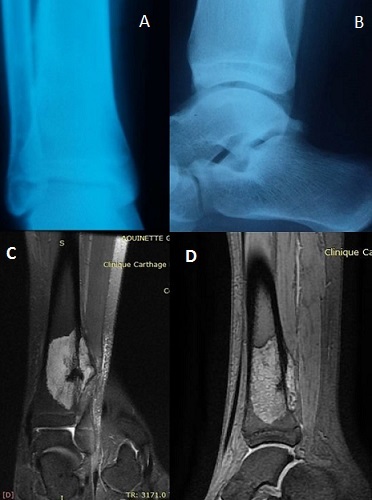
(A,B) plain radiographs showing a metaphyseal osteolytic lesion of the distal left tibia; (C,D) MRI, an extension to the adjacent soft tissue and no epiphyseal involvement
